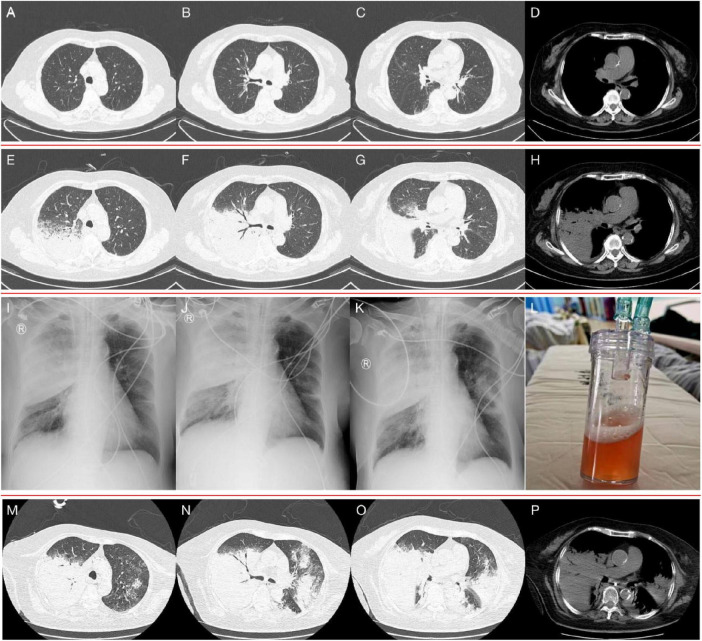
(A–D) Chest CT on admission, showed emphysema and slight thickening of the bronchial wall. (E–H) Chest CT on hospital day 16, showed a new sizeable consolidation in the upper right lung with blurred borders, a bronchial inflation sign, slightly patchy exudative opacity in the right middle lobe, and a small amount of pleural effusion on the right chest. (I–K) Bedside chest radiography on hospital day 18, 20, and 22, respectively, showed gradual enlargement of consolidation in the upper right lung and the development of new patchy exudative opacity in the upper left lung. (L) orange-colored BALF was collected at RB2 on hospital day 22. (M–P) Chest CT on hospital day 24, showed significant progression of the lesions and exudative consolidation in multiple lobes of both lungs.
